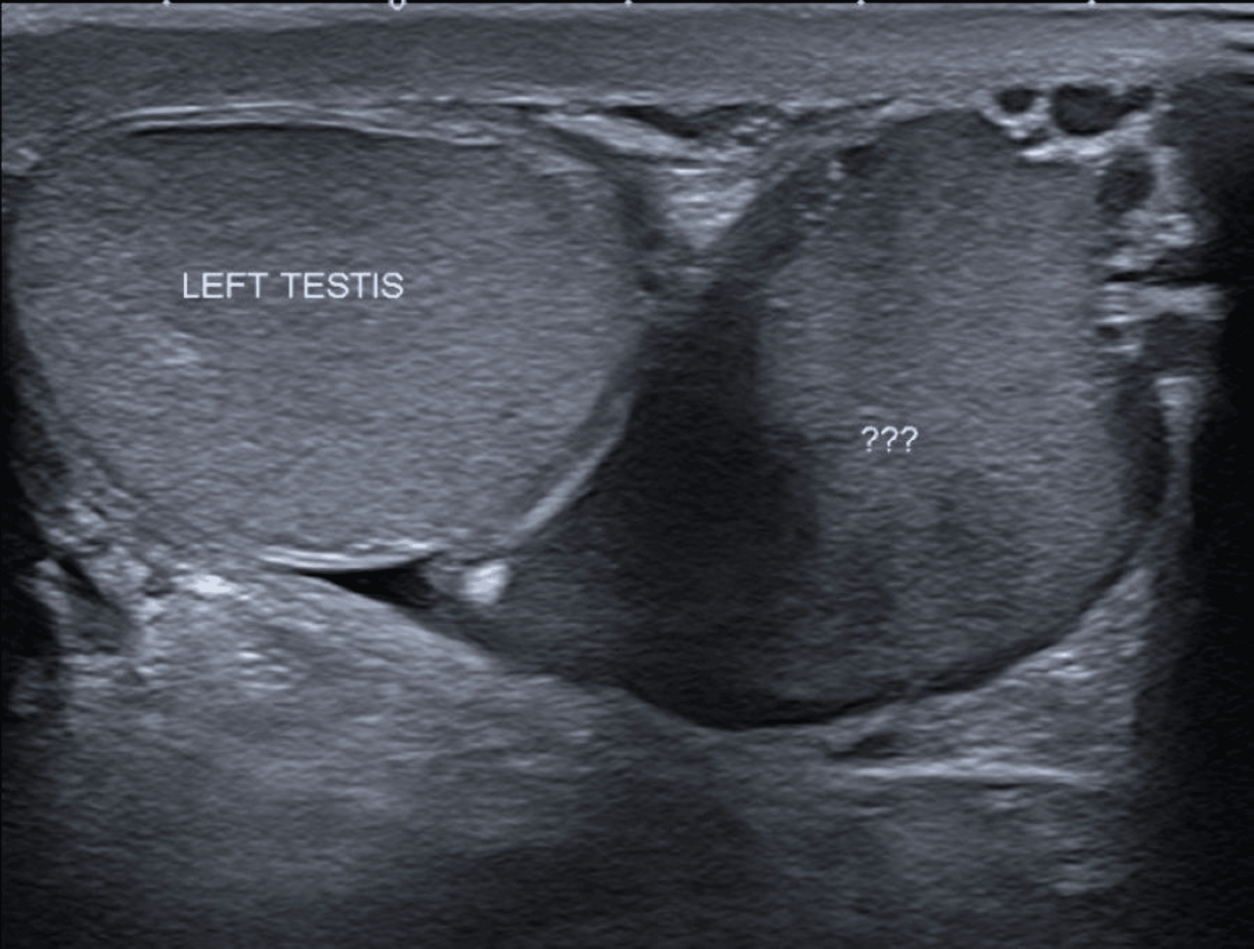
Case 1: The repeat ultrasound scan requested for this patient shows the initial diagnostic uncertainty. At this stage, the tumour measured 4 cm in width. The question marks (???) were inserted by the radiologist during imaging. They indicate the region that was subsequently diagnosed as liposarcoma of the spermatic cord, although this was not known at the time of imaging.
